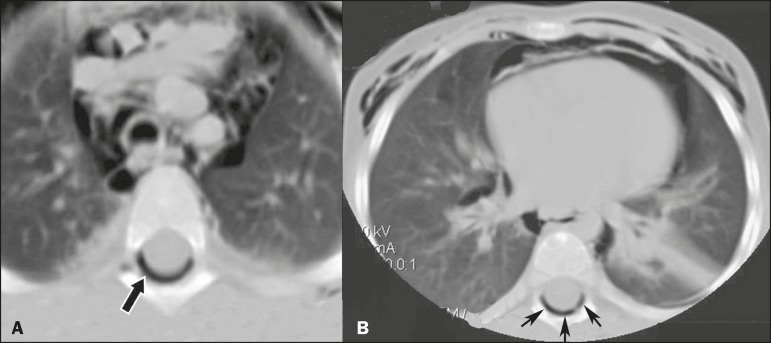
Chest CT scan, at the level of the upper (A) and lower (B) lobes, showing
